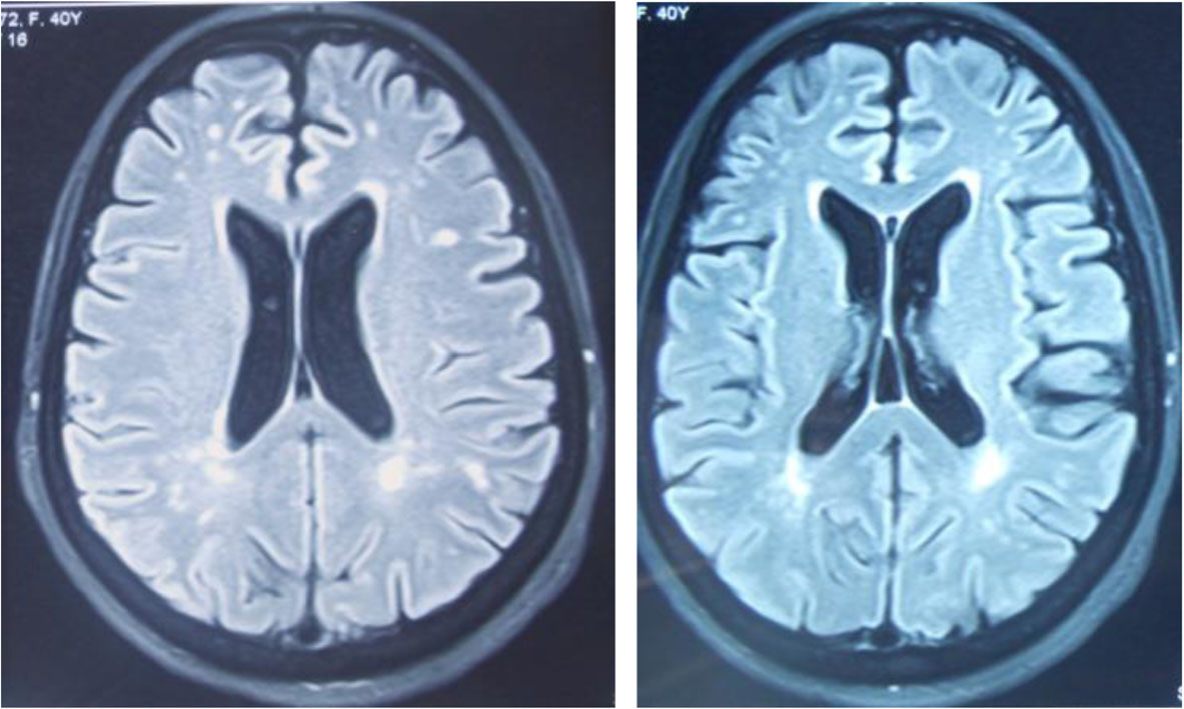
Brain MRI FLAIR sequences showing bilateral hyperintensities, affecting periventricular regions predominantly in the subcortical white matter
